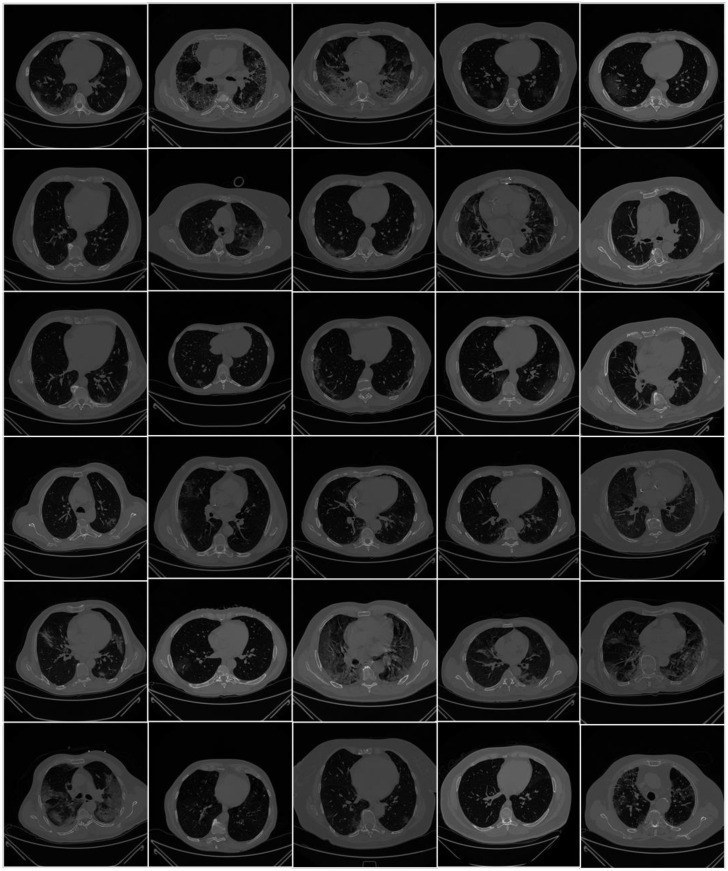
Raw lung COVID-19 CT images were taken from different patients in the database.
